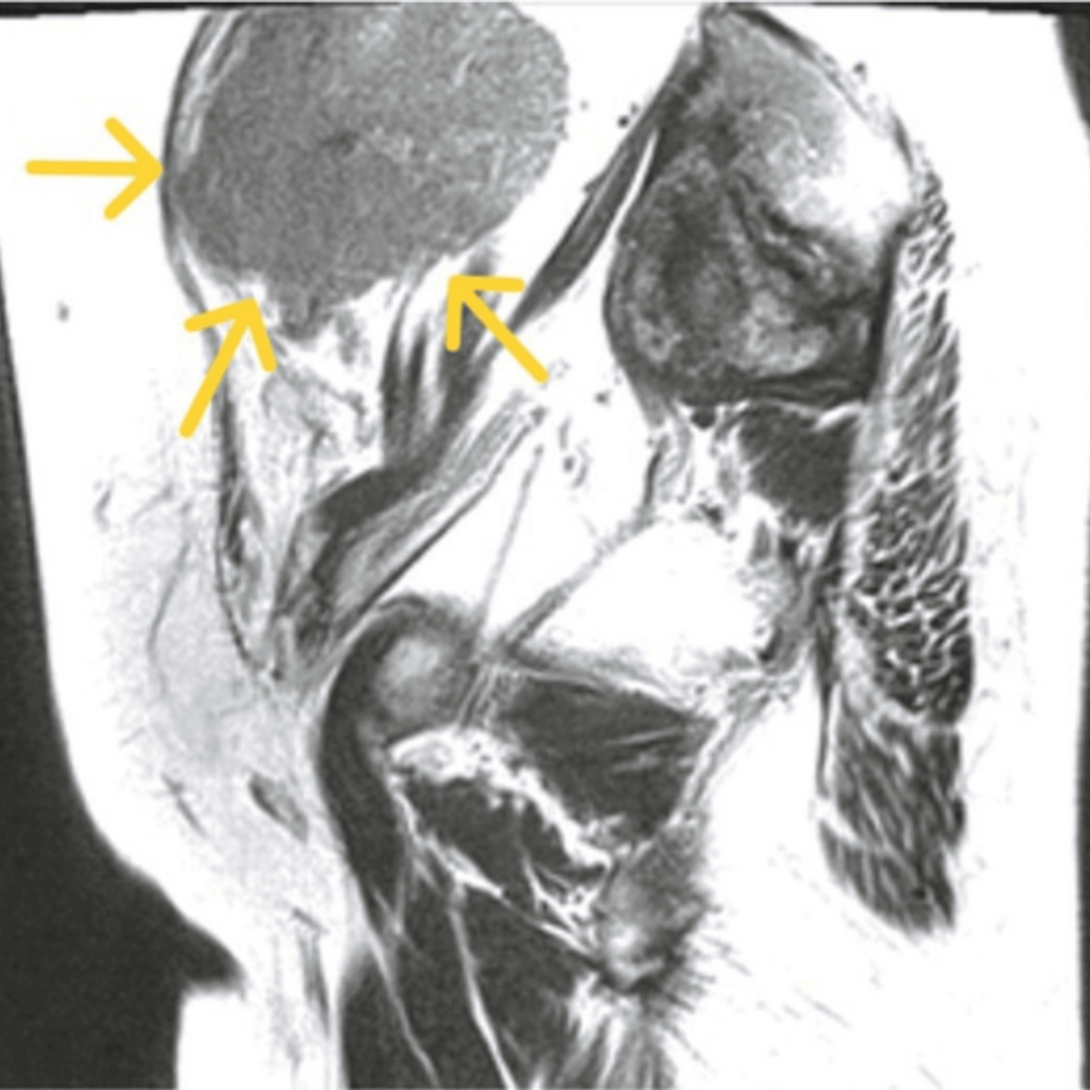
T1-weighted MRI scan. MRI demonstrating a 76 × 56 mm mass (yellow arrow) with slightly higher T2 signal intensity than the myometrium and low T1 signal intensity.
